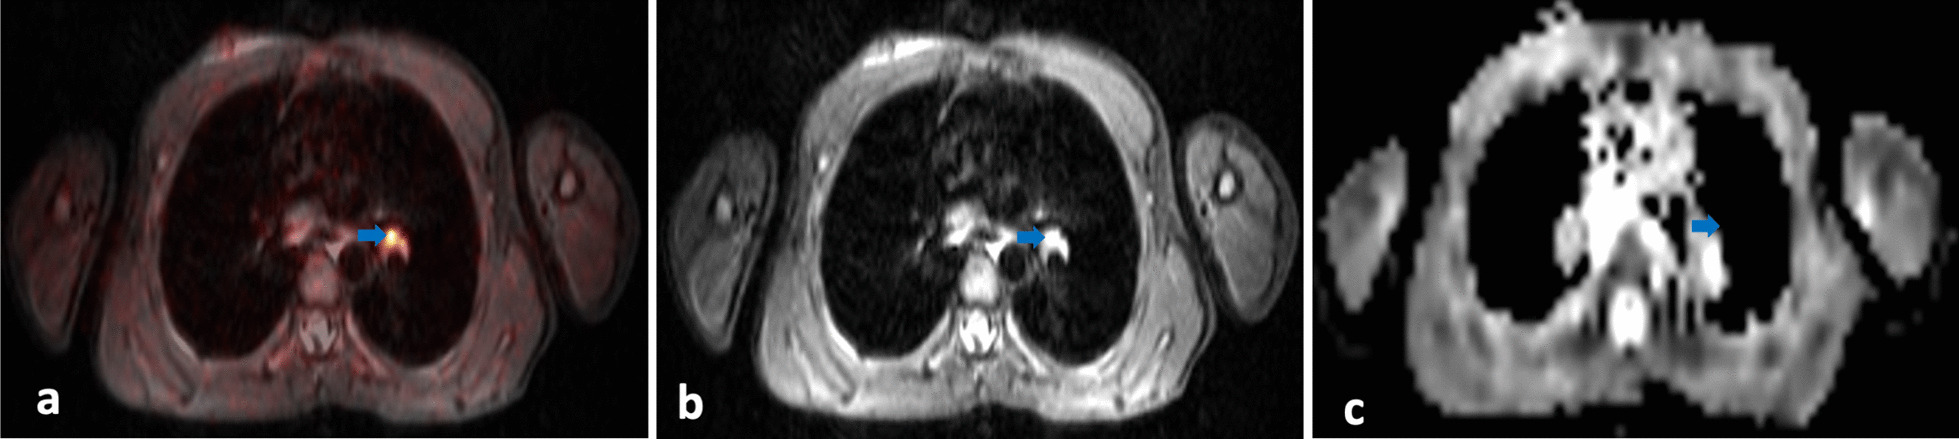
a–c [^18^F]-FDG-PET/MR image (a), T2-TIRM image (b) and DWI (c) of the thorax. The left hilar lymph node (blue arrow) has increased glucose metabolism (SUV_max_ 3.3) (a) but is small (0.9 × 0.7 cm) (b) and without diffusion restriction (ADC: 3800 × 10^–6^ mm^2^/s) (c)
